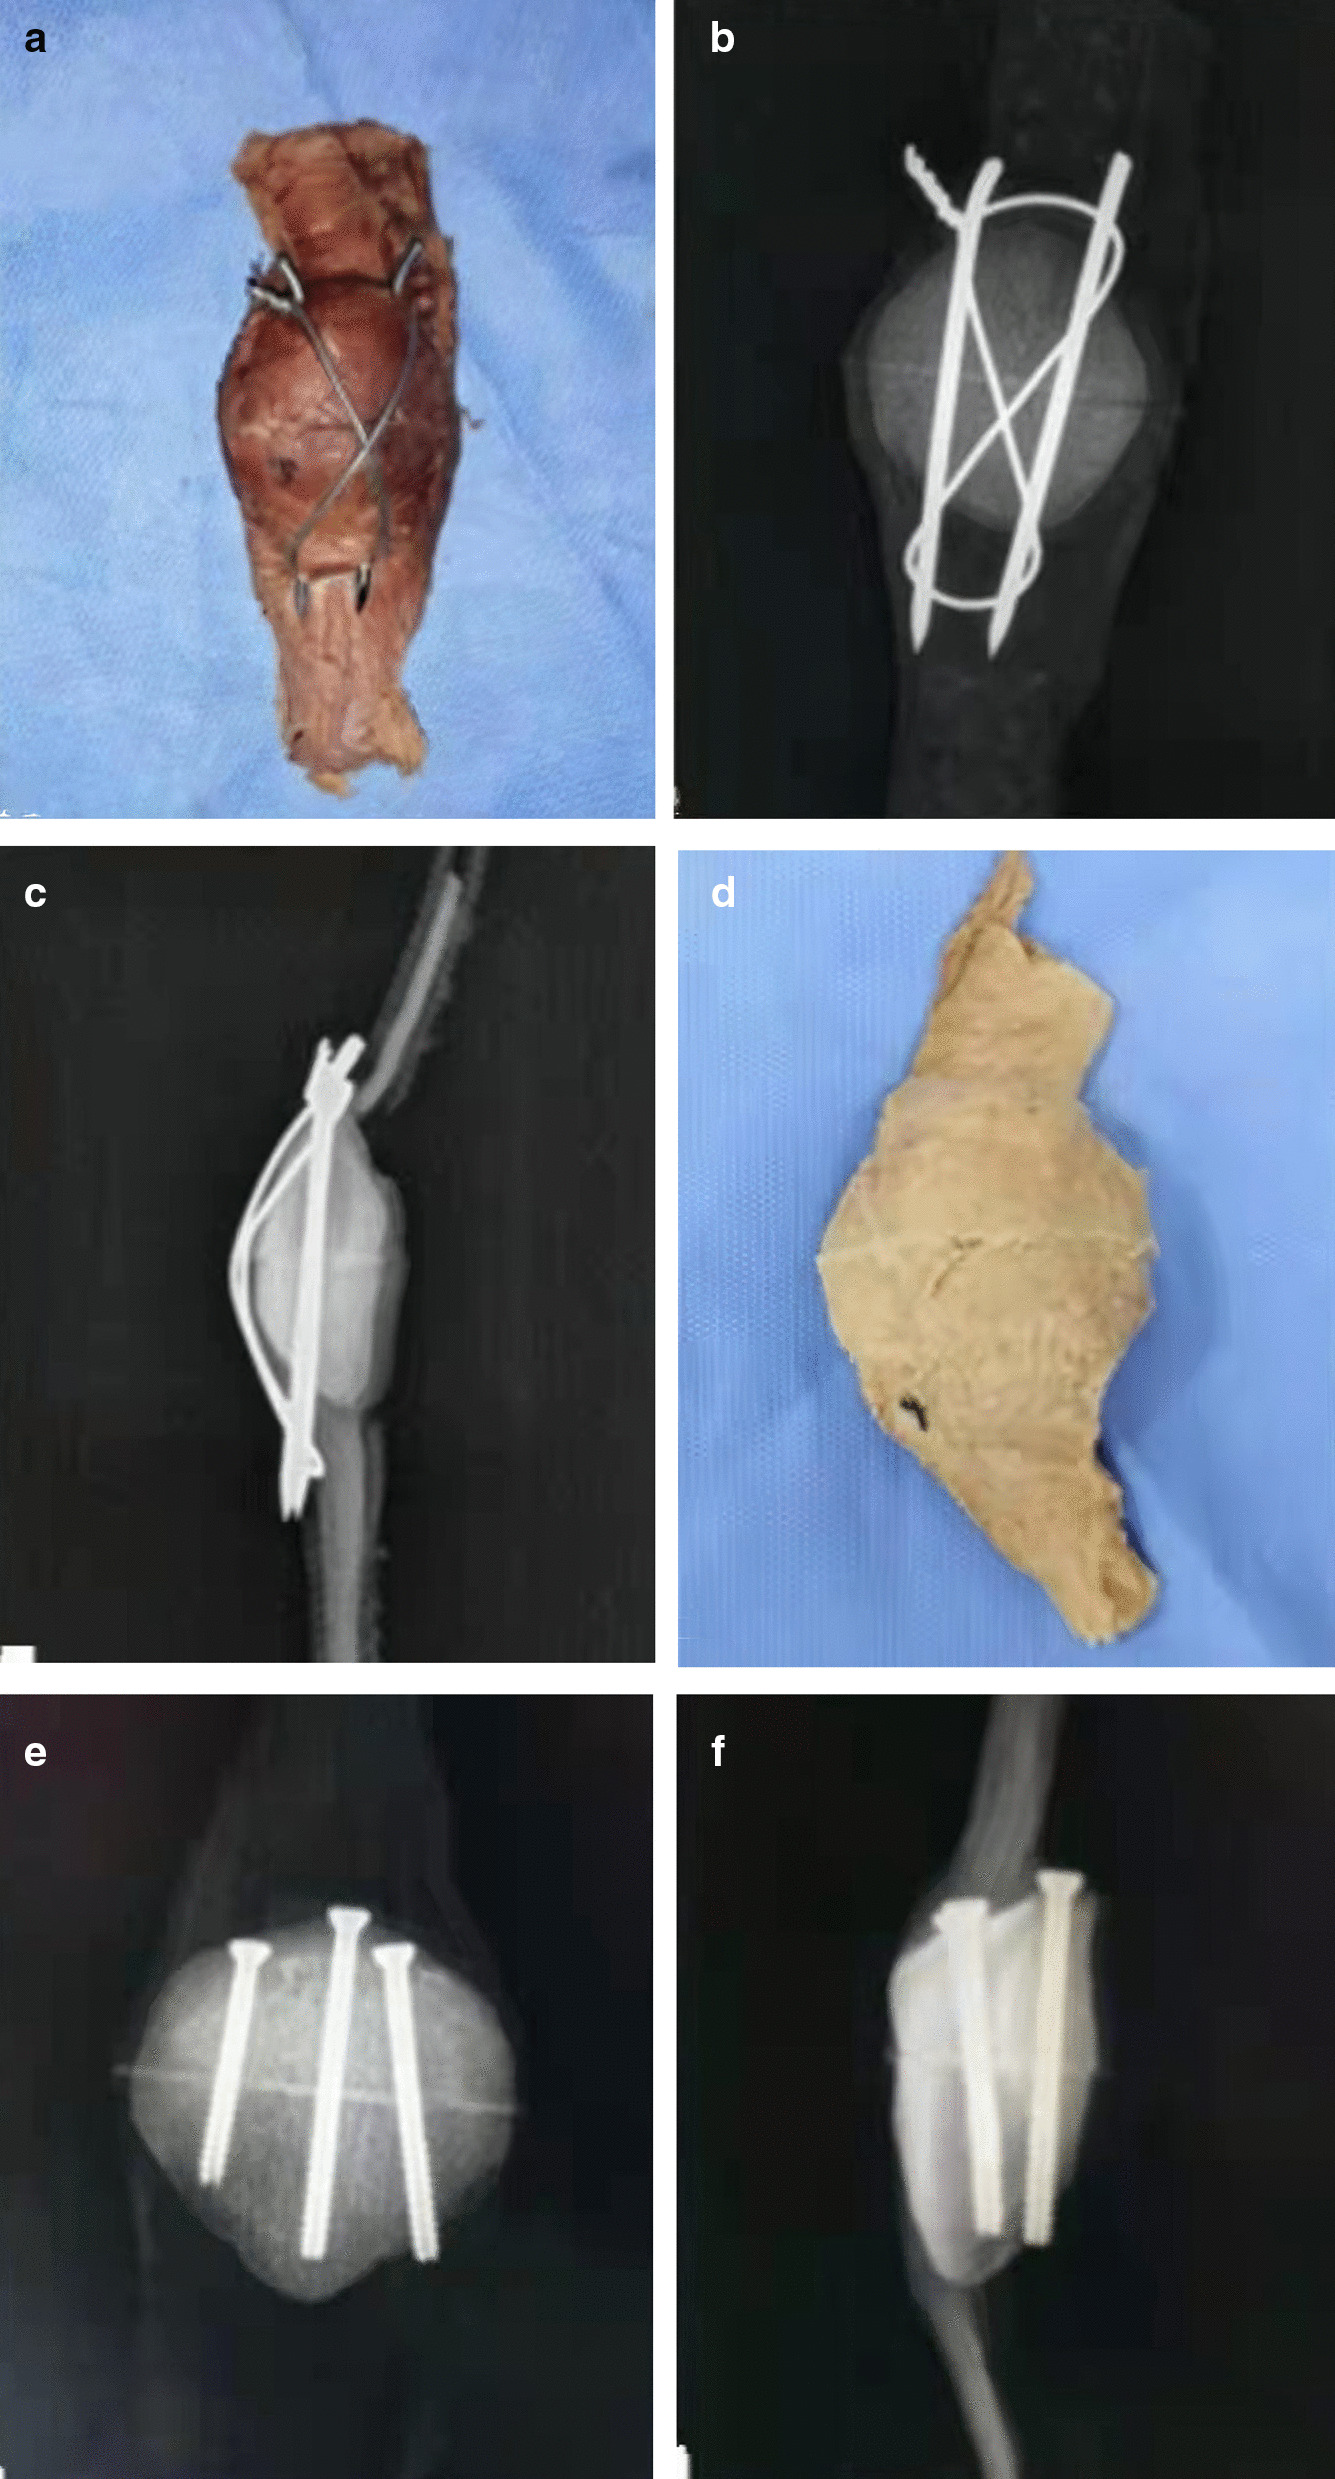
A The K-wire tension band technique was performed: B anterior–posterior (AP) and C lateral radiograph; D the three cannulated screws' technique was shown: E AP and F lateral radiograph
